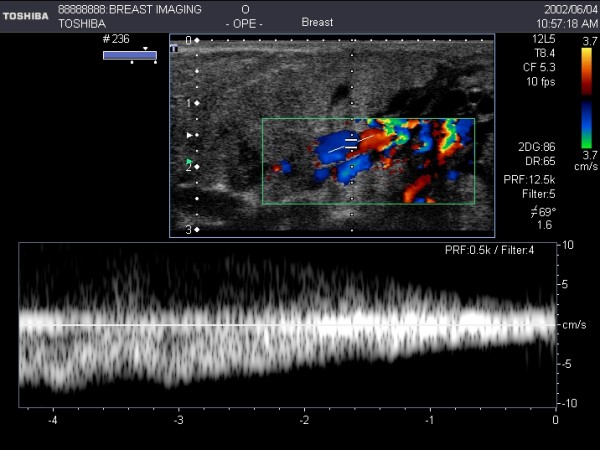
Colour flow Doppler imaging of milk flow within multiple ducts at milk ejection. Colour Doppler detected the movement of milk within the milk duct at milk ejection. Using pulsed wave Doppler the velocity of milk flow can be measured. Measurement of milk flow within a milk duct is difficult due to Doppler angle, the transient nature of milk ejection and low velocity milk flow.
